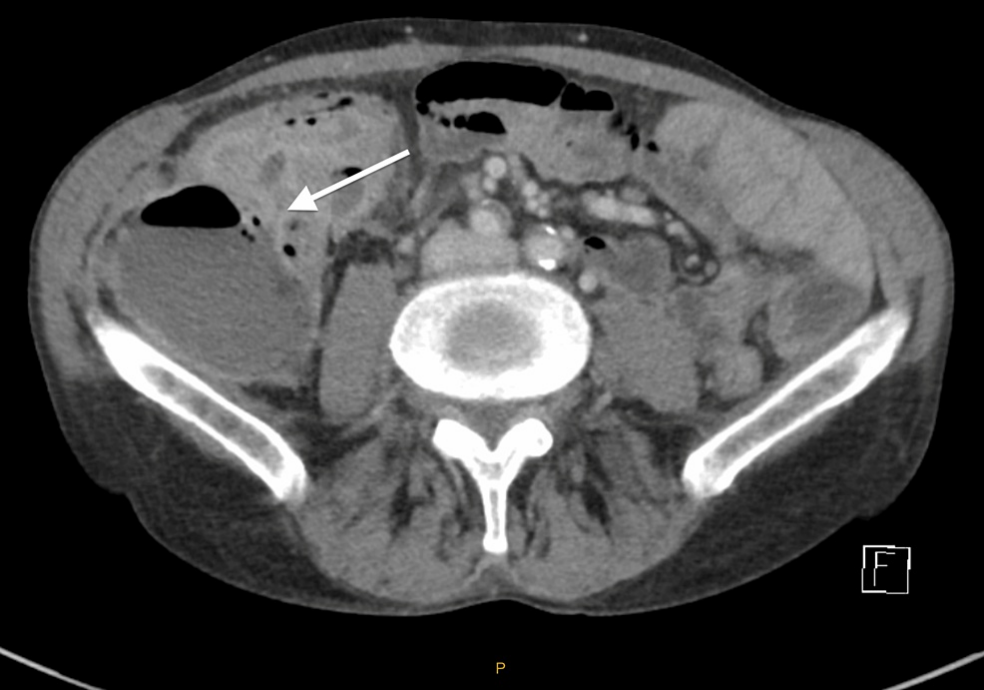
CT abdomen showing intra-abdominal collection marked by a white arrow
